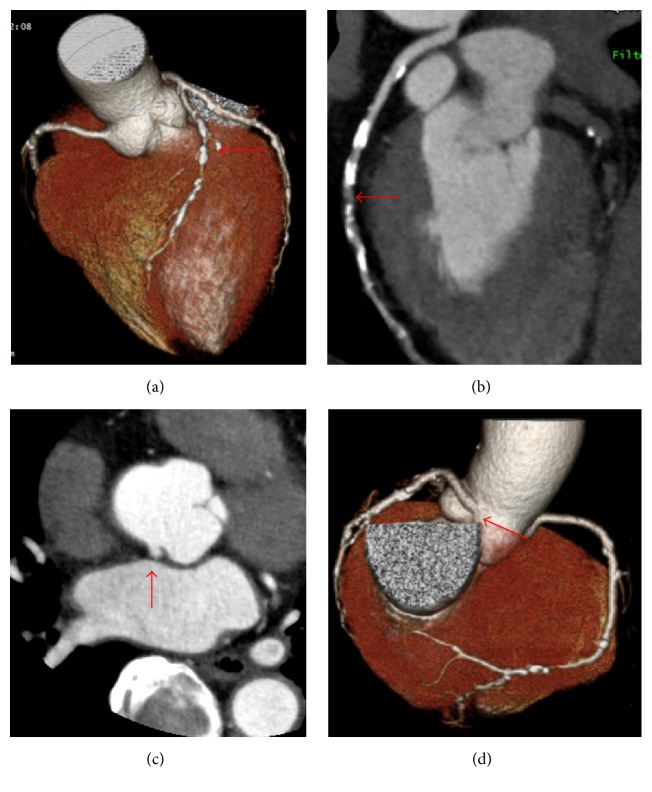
(a) CT (3D view) showing stenosis of the mid-LAD artery (indicated by the red arrow). (b) Multiplanar reconstruction view of the LAD artery (CT). The red arrow shows stenosis of the mid-LAD artery. (c) Red arrow showing arising ostium of the LCA (sectional view, CT). (d) Red arrow showing arising ostium of the LCA (3D view, CT).
